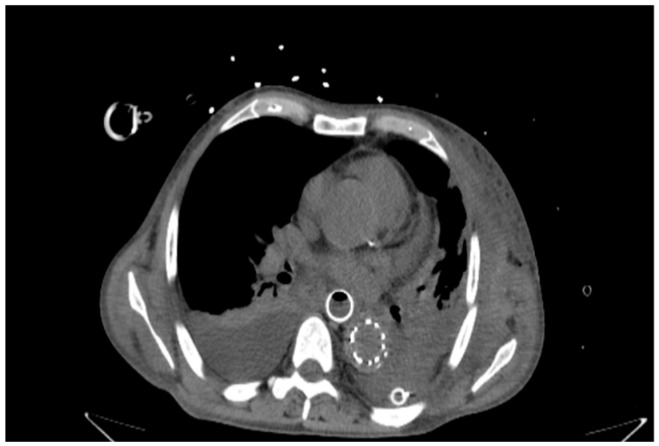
CT scan showing the postoperative setup after emergency treatment. The image displays the fully covered esophageal stent in place and the thoracic aortic endograft.
